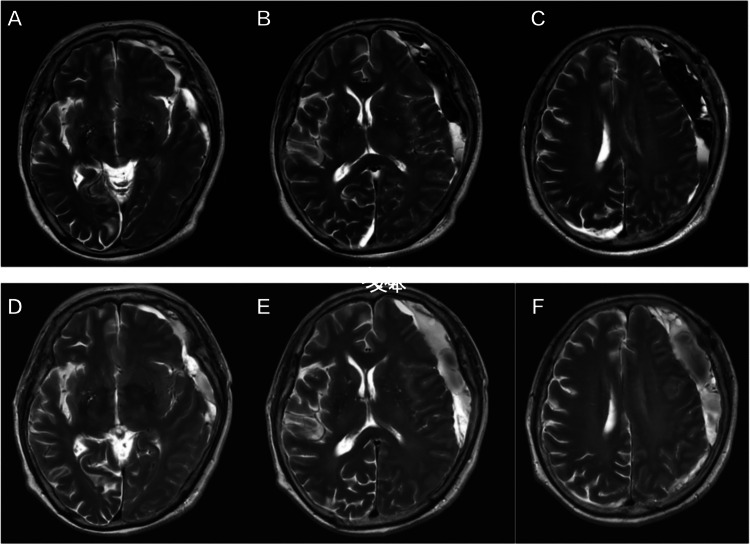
Axial MRI images after the first operation. Upper row (A, B, C): Postoperative MRI (day seven) indicating a massive recurrent left chronic subdural hematoma (cSDH) with midline shift. Lower row (D, E, F): Postoperative MRI (day 14) revealing the recurrent left cSDH with no improvement in the midline shift.
